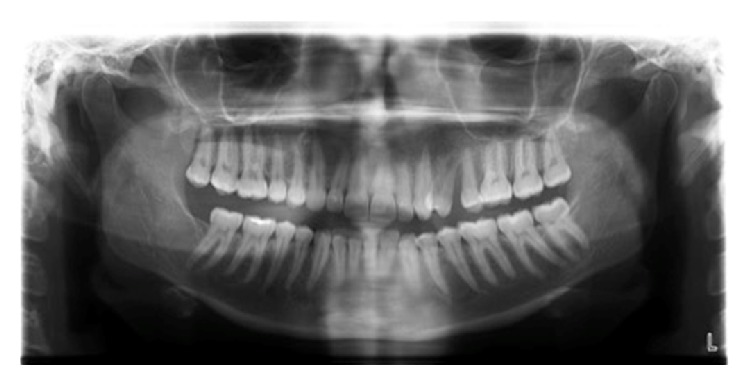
Panoramic radiograph at initial presentation. Interproximal bone loss can be appreciated between teeth numbers 24 and 25 (international nomenclature) and tooth number 24 is noted to be mesially displaced.
